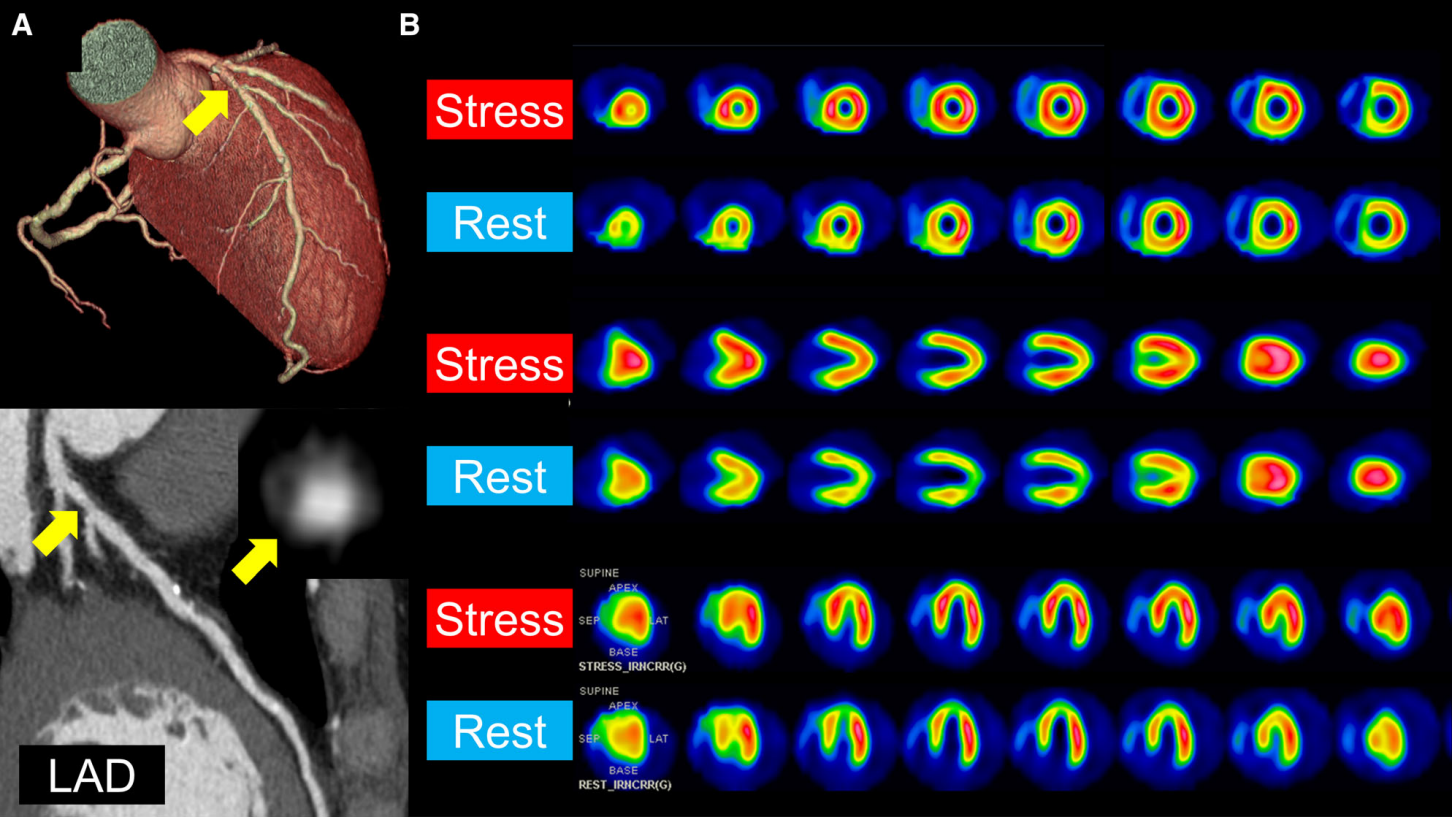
(A) Coronary computed tomography angiography revealed a mild stenosis (yellow arrows) with low-density non-calcified plaque in the proximal left anterior descending coronary artery (LAD). (B) Dipyridamole stress and rest ^99m^Tc-tetrofosmin myocardial perfusion imaging (MPI). Stress MPI (300 MBq of ^99m^Tc-tetrofosmin) showed no perfusion defect, whereas rest MPI (900 MBq of ^99m^Tc-tetrofosmin) 2 hours after the stress test showed reduced uptake (reverse redistribution) in the LAD territory
